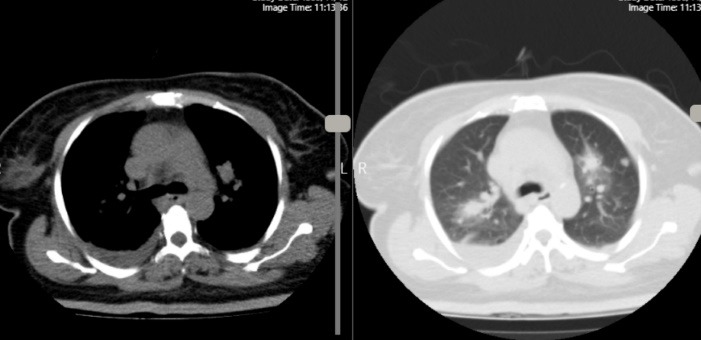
According to modified Duke Criteria for infective endocarditis, the patient had one major and two minor criteria for IE. So the patient underwent experimental treatment of infective endocarditis with Meropenem and Vancomycine but despite anti-biotic therapy, the patient’s fever persisted. In lab data, WBC count of 2100 and ESR level of 81 were found. She underwent echocardiography again and no change in size of vegetation or no evidence of abscess formation was seen. Considering fever and vegetation size, the patient was scheduled for surgical excision of vegetation on TV with valve replacement. Intraoperative observation reported large vegetation associated with destructed valve; so it was replaced by a mechanical bileaflet prosthetic valve.
